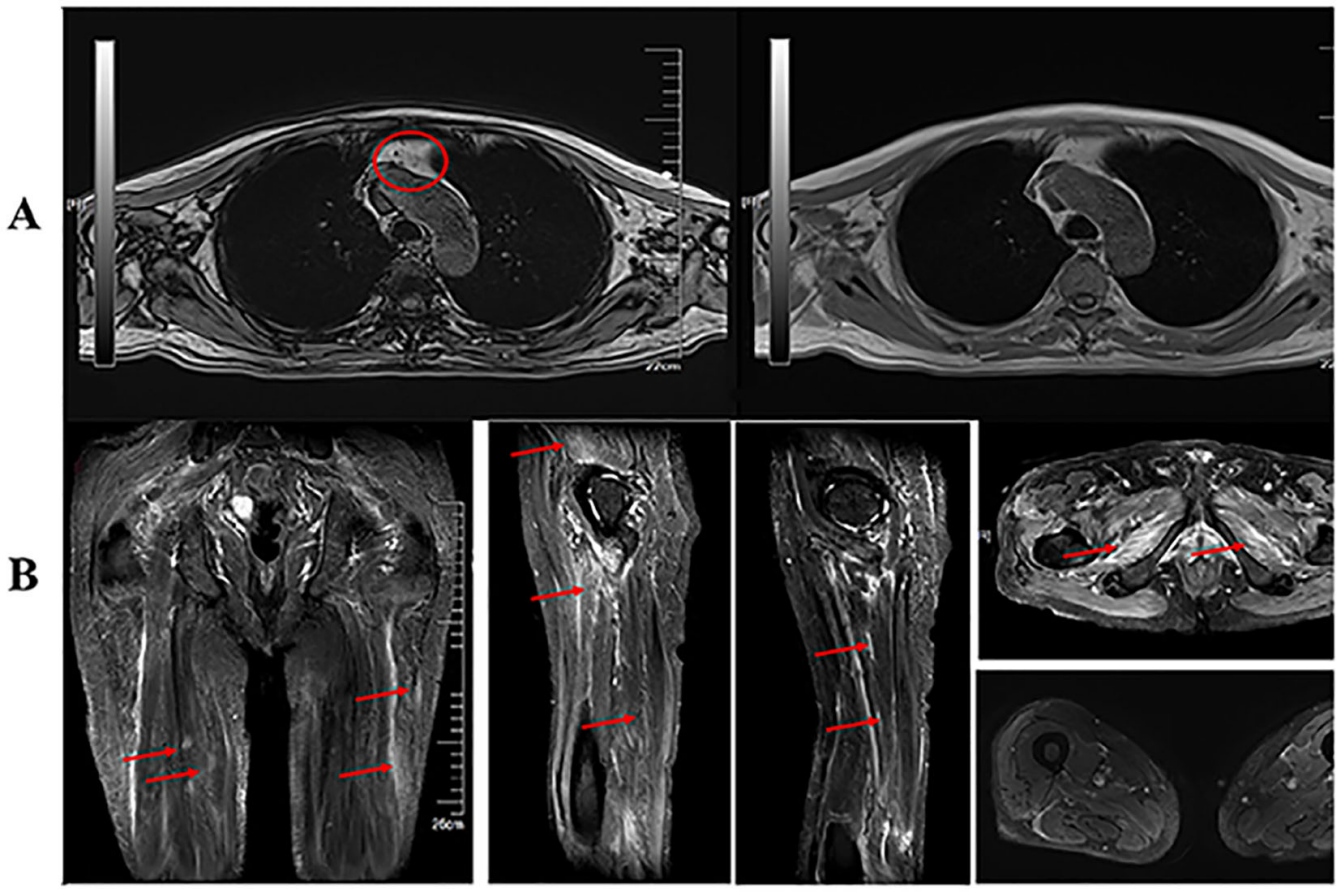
(A) Magnetic resonance imaging (MRI) of the patient’s thymus revealed a slight narrowing of the antiphase area in the mediastinum thymus region, and no abnormalities were seen on enhanced CT. (B) There were no abnormal lymph nodes in the hilum or mediastinum. This was considered to be thymic hyperplasia. Peripelvic floor muscles, bilateral buttocks and bilateral thighs showed diffuse hyperintense T2 fat-suppression signals. Some of the muscles were mildly atrophied.
